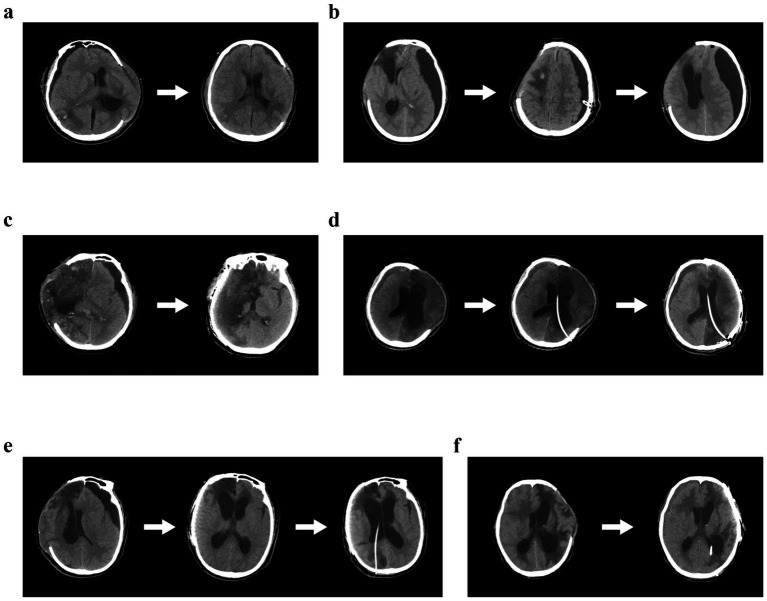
Radiographic progression of cSDE and PTH following treatment. (a) Following conservative treatment, both the cSDE and PTH showed resolution. (b) After burr hole drainage of the cSDE, the effusion improved but it may recur without continuous drainage. (c) After cranioplasty, the cSDE resolved, and no PTH developed. (d) VP shunt placement was initially performed to address the hydrocephalus, followed by cranioplasty. (e) Following cranioplasty, the cSDE resolved, but the PTH subsequently worsened, requiring VP shunt placement. (f) After conservative treatment, the cSDE resolved, and the patient simultaneously underwent VP shunt placement and cranioplasty.
